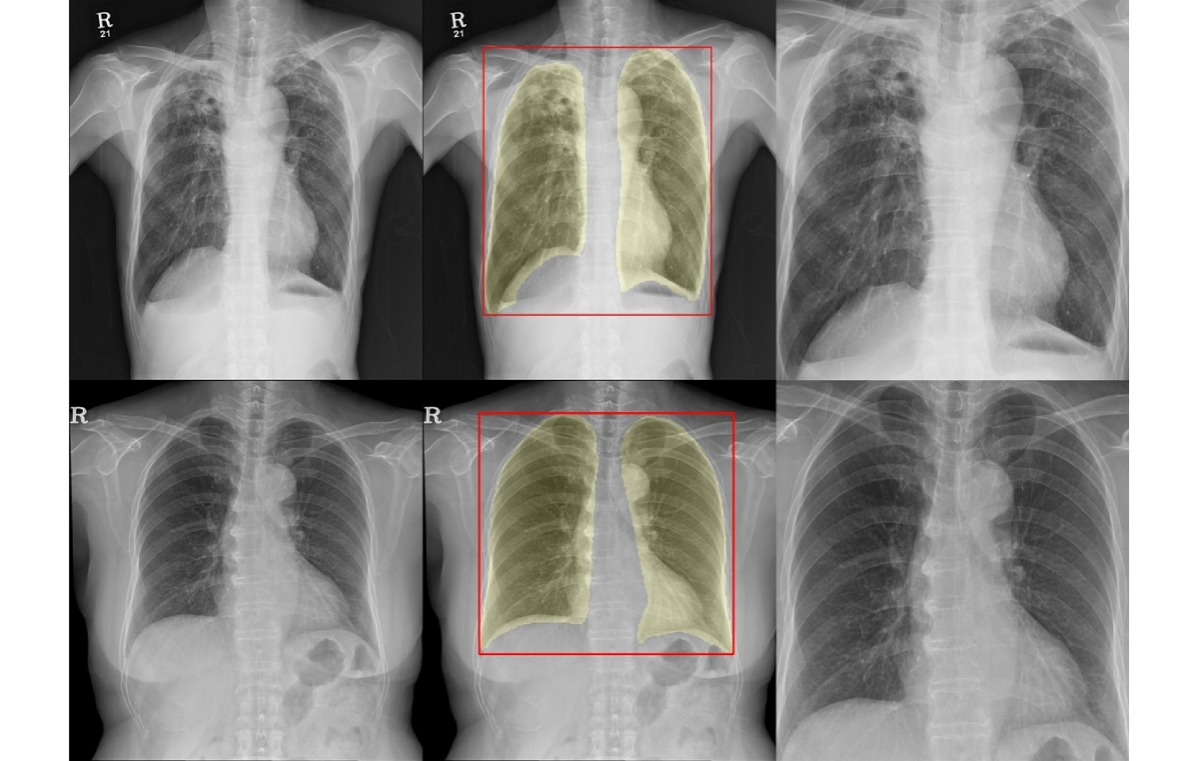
Lung area segmentation and cropping in chest radiographs to enhance tuberculosis infectivity assessment. Left: original chest radiographs; center: segmented and cropped lung area; right: chest radiographs used for analysis.
